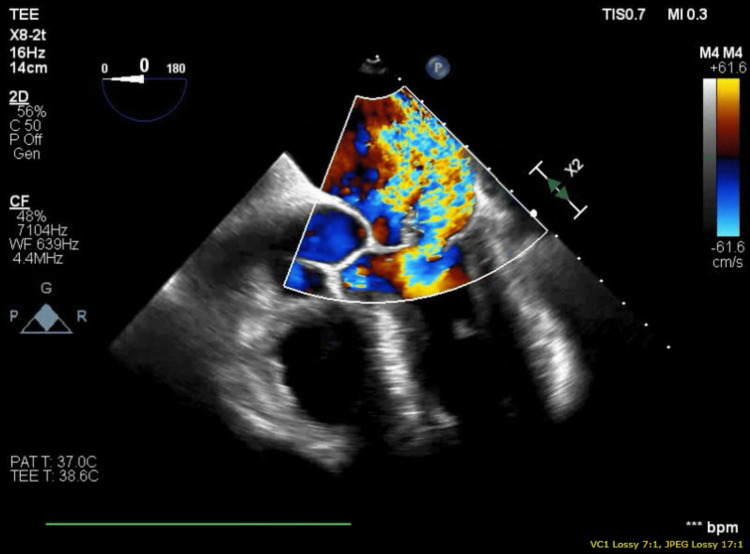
Transesophageal echocardiogram mid-esophageal five-chamber view showing severe mitral regurgitation
